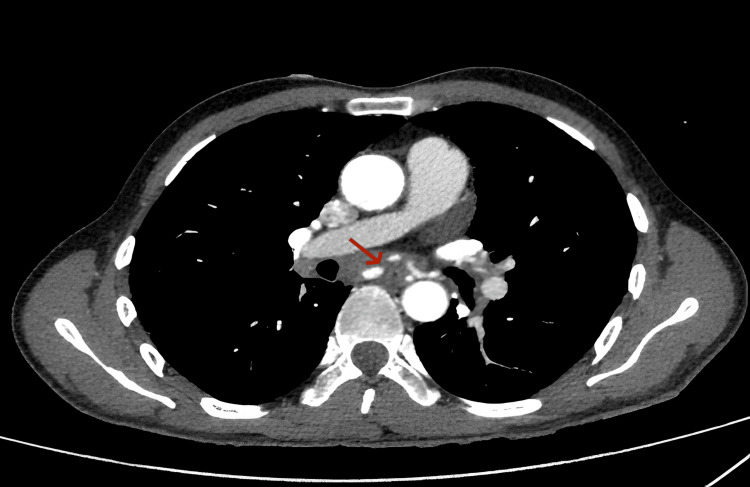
CT scan with injection showing bleeding (red arrow).
